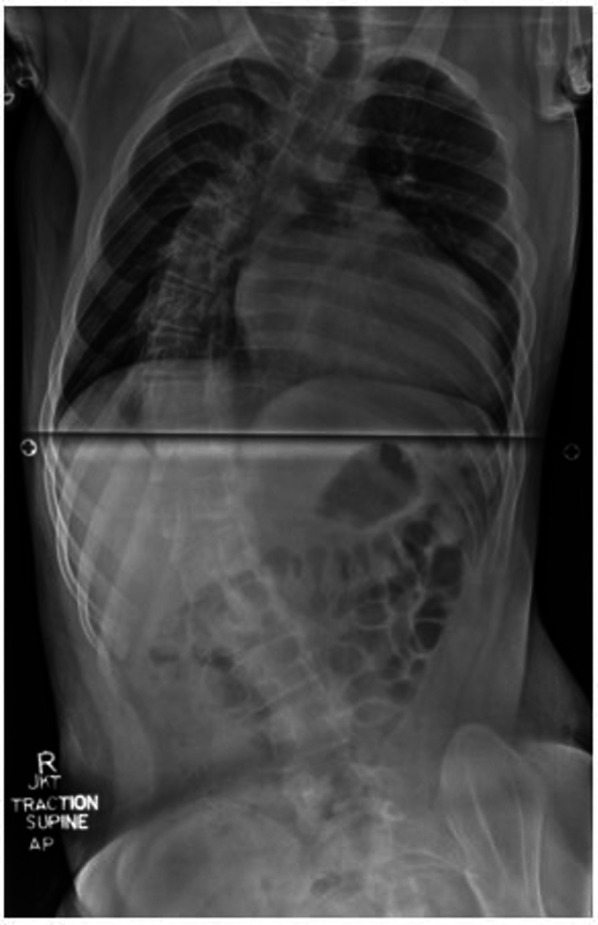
Full spine X-ray demonstrating severe long segment right thoracolumbar rotatory scoliosis.
